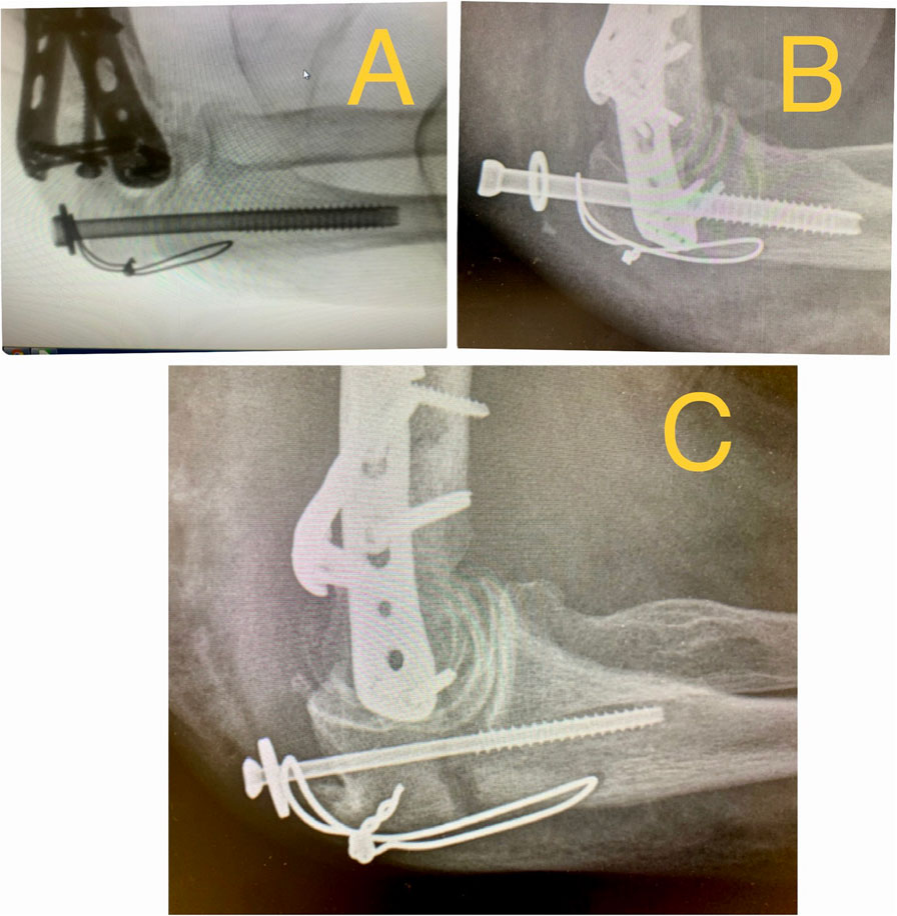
a Lateral intraoperative X-ray of 74 year old woman who presented a conminuted supraintercondylear fracture of the humerus, fixed with two plates and a 6′5 mm thick and 60 mm long screw with a washer and a cerclage for the olecranon osteotomy. The screw is not long enough to grip the cortical of the ulna canal diaphysis. b Same case; X-ray 5 weeks after the surgery with acute failure of the osteosynthesis of the olecranon osteotomy. In this case, we waited 4 more weeks until the osteotomy consolidation and then we removed the screw and cerclage. c Lateral X-ray of a 68 year old man with a supraintercondylear fracture treated with two plates in the humerus and a 4′5 mm thick and 42 mm long screw and a cerclage for the olecranon osteotomy. In this case an acute loss of reduction of the fixation of the osteotomy appeared, with a gap of more than 2 mm. The screw is not long neither thick enough to grip the cortical of the ulna canal diaphysis and provide enough stability
